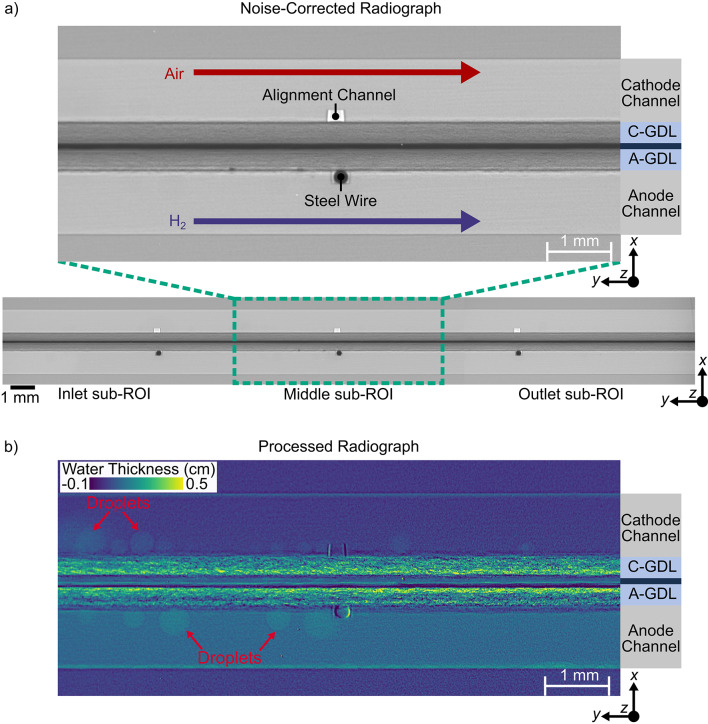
a Dark, Flat, and Intensity corrected radiograph of the middle sub-ROI of the fuel cell assembly at the 180° angle, and b Resulting radiograph after reference subtraction and application of the Beer-Lambert law to obtain water thickness values in the entire assembly. A high amount of water can be observed in both the anode and cathode GDLs. Water droplets (annotated in red) can also be clearly seen forming from the GDL into the channels of both anode and cathode. Additionally, the entire anode is brighter than the cathode channel, indicating that at least one anode channel was completely full of liquid water during operation.
